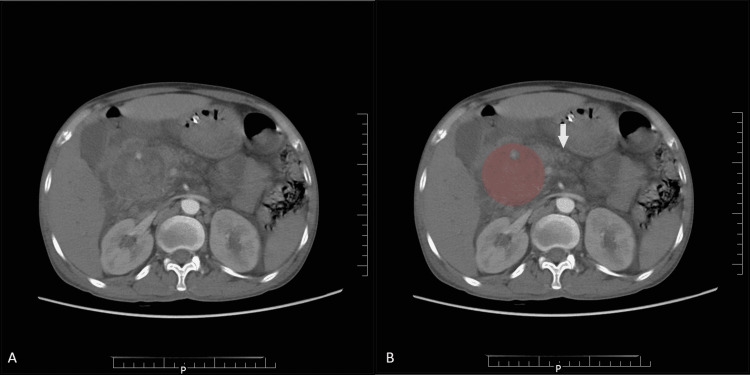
Contrast-enhanced CT axial sections of the abdomen CT: Computed Tomography Images A and B show pseudocyst of the pancreas measuring 55 x 54 mm (red in B) and hematoma (green in B) located posteriorly to the pancreas (arrow in B).
